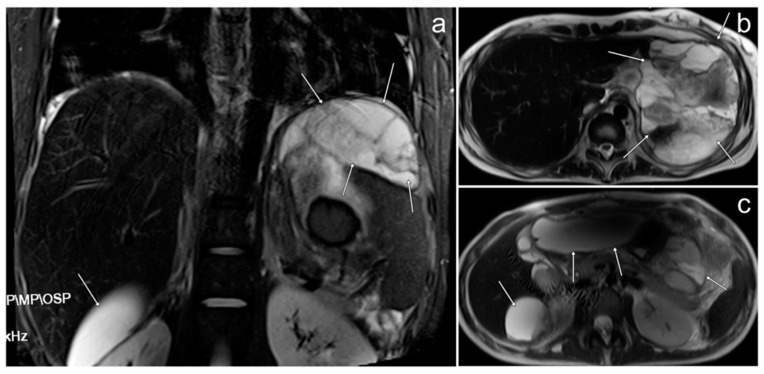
The MRI of the peritoneal metastases of ovarian angiosarcoma (a–c, arrows). (a)—STIR; (b,c)—FSE/T2 (images courtesy of Dr. M. I. Furmanek, Medical University of Warsaw).
